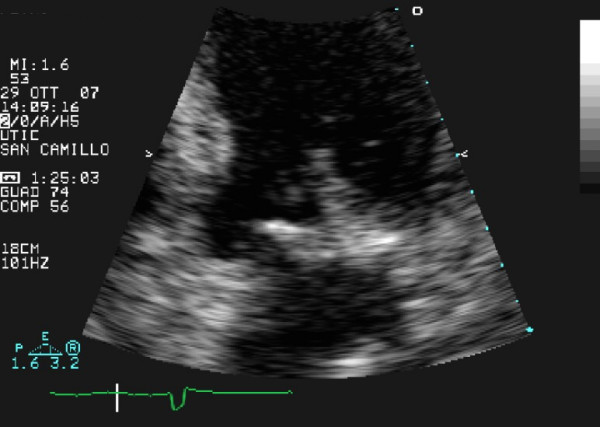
Two-dimensional echocardiogram, apical 4-chamber view (detail): vegetation is shown at a higher magnification.
